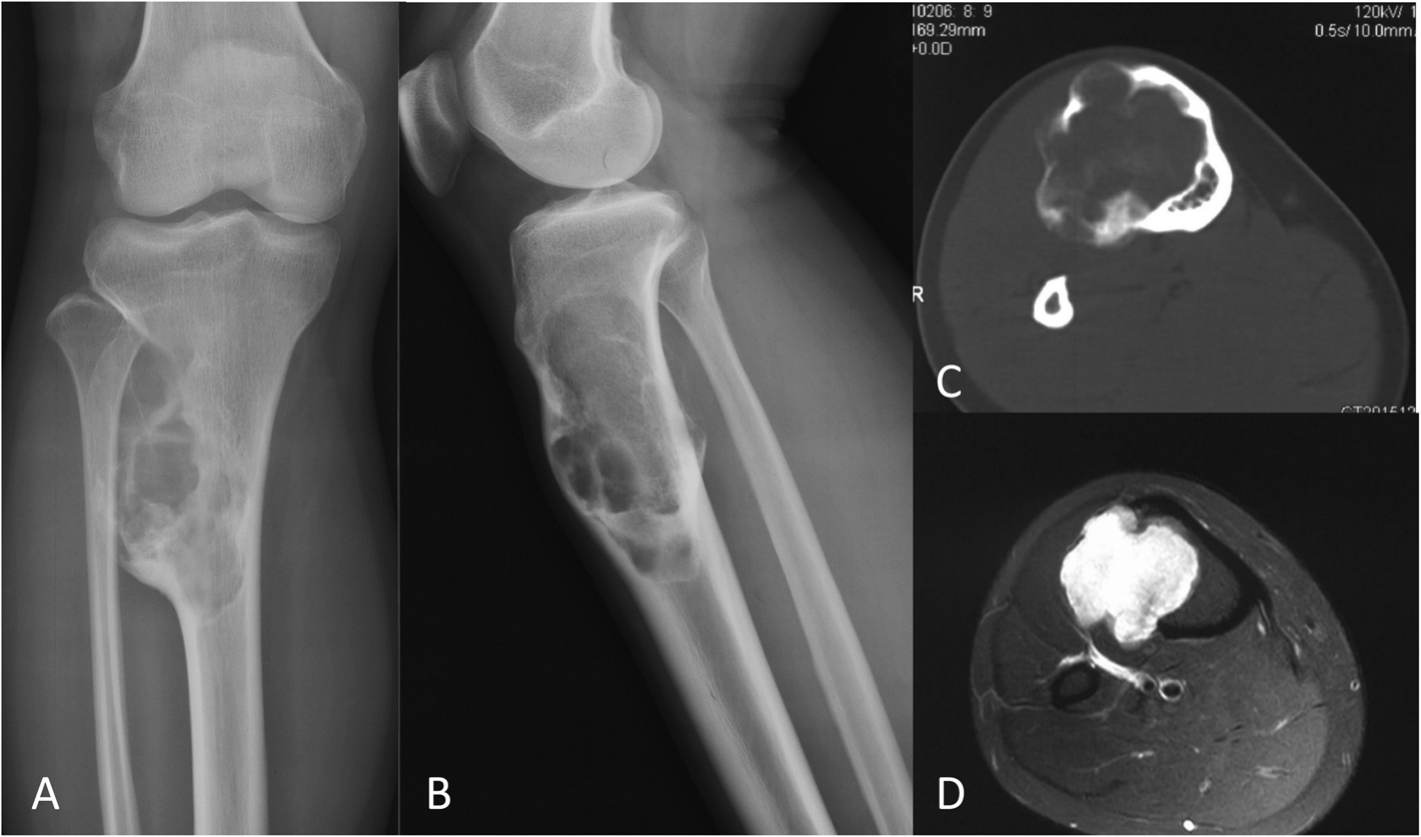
Representative images of osteofibrous dysplasia-like adamantinoma. Radiographs showing a anterior-posterior and b lateral views of the tumor in the tibial shaft. c CT image showing the extent of the tumor in the cortex. d MRI showing the extent of the tumor
